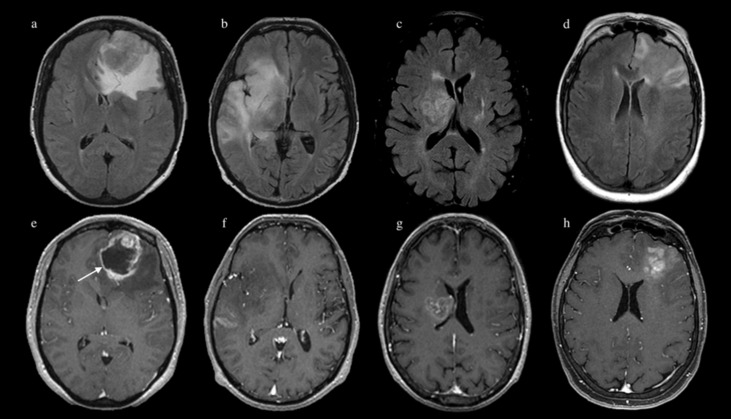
FLAIR images (above) and post-contrast MPRAGE images (below) of four patients with glioblastoma multiforme (GBM) from our cohort. Patient 1 (a,e) presented an expansile left frontal lobe GBM with typical features of rim-enhancement and central necrosis (arrow on image e); pathology confirmed isocitrate dehydrogenase (IDH)-wildtype. Patient 2 (b,f) presented a diffuse non-enhancing infiltrative GBM of the right temporo-insular region; pathology confirmed IDH mutation. Patient 3 (c,g) and 4 (d,h) demonstrated ‘borderline’ features, not typical for any IDH status; pathology confirmed IDH mutation for patient 3 and IDH-wild type for patient 4.
